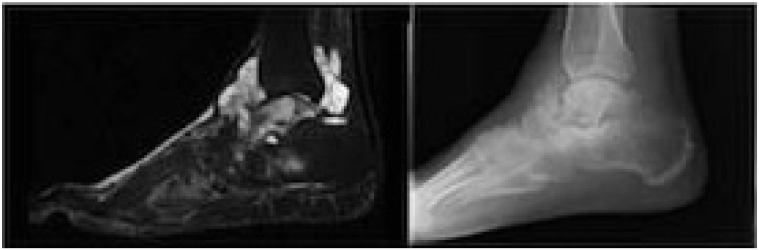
Synovial chondrosarcoma in the setting of synovial chondromatosis 47 year-old male with two prior ankle injuries and pain over the past 3 years now presenting with recent progression of pain and swelling. Initial biopsy was consistent with grade II/III chondrosarcoma, confirming the radiographic appearance (MRI, A; Plain XR, B) and clinical presentation. He underwent below-knee amputation.
